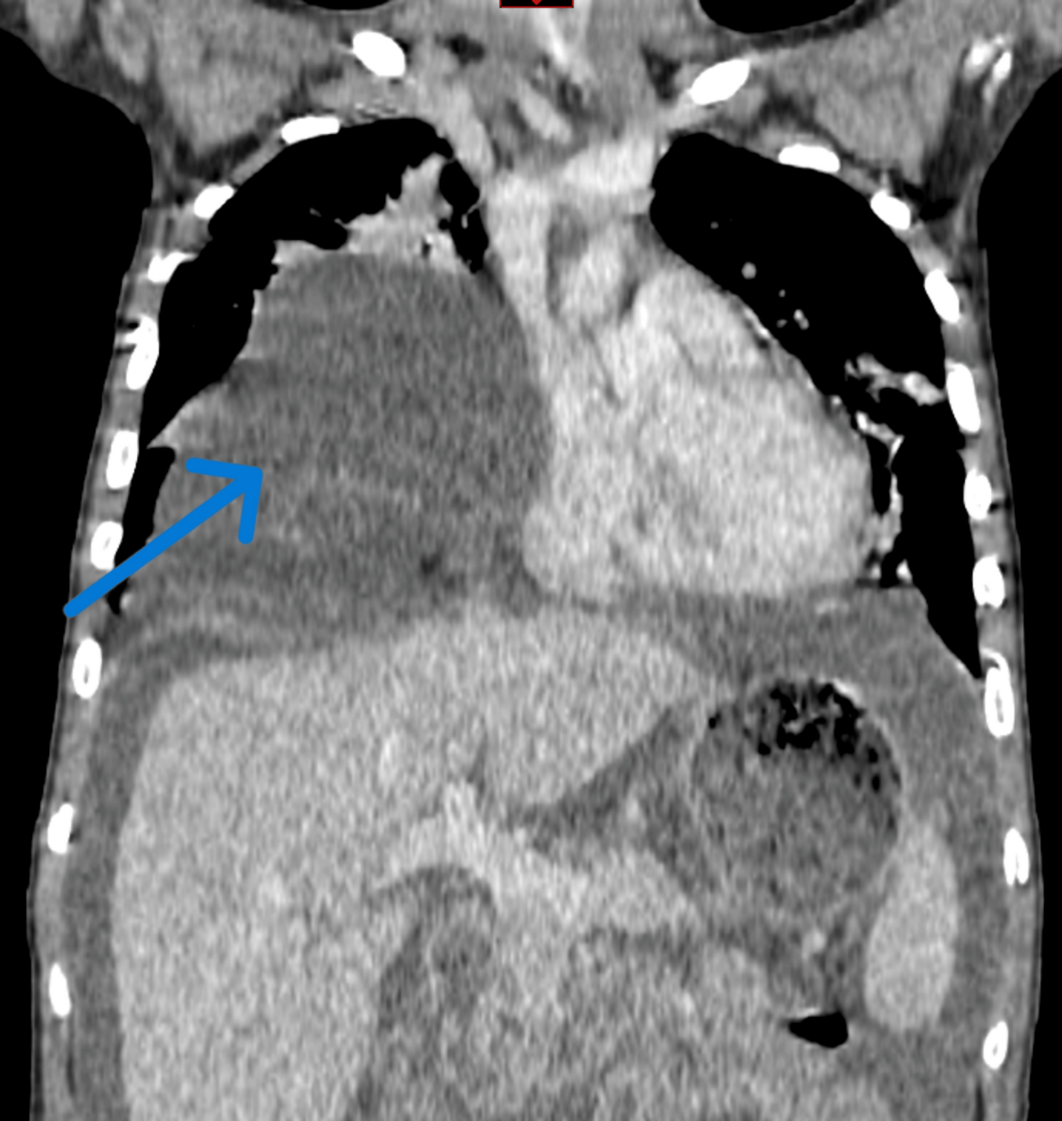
Coronal section of the thoracoabdominal CT scan showing the hernia (arrow) with slight displacement of left-sided structures, including the heart CT: computed tomography
